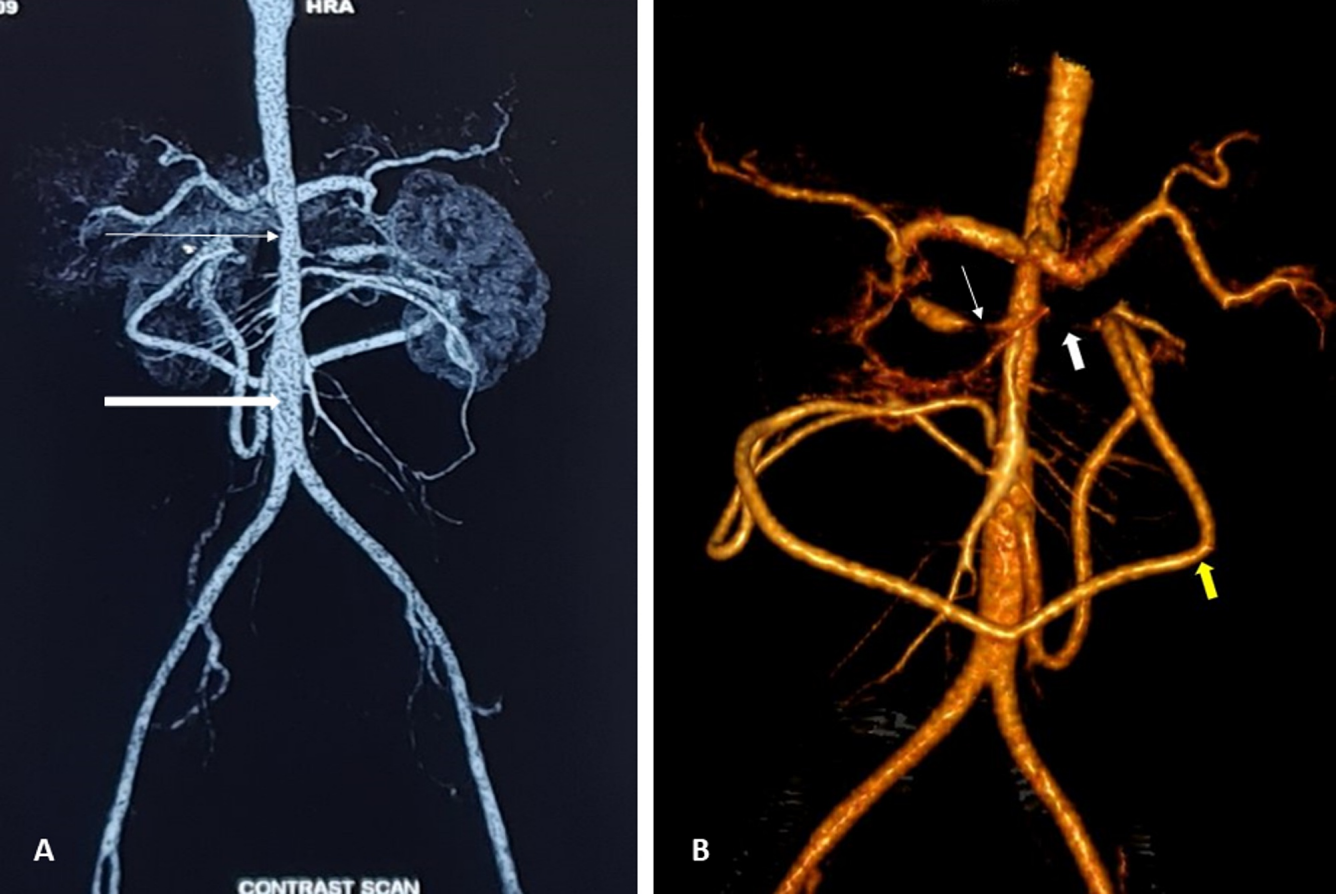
CECT arterial-phase image. (A) Significant luminal narrowing of the distal descending thoracic aorta (thin white arrow) with poststenotic dilatation (thick white arrow). (B) Three-dimensional volume-rendered image showing grossly stenosed right renal artery (thin white arrow) with poststenotic dilatation. The proximal left renal artery is not visualized (thick white arrow) due to severe narrowing. The inferior mesenteric artery is hypertrophied (yellow arrow) and anastomosing with the branches of the superior mesenteric artery. CECT, contrast-enhanced computed tomography
